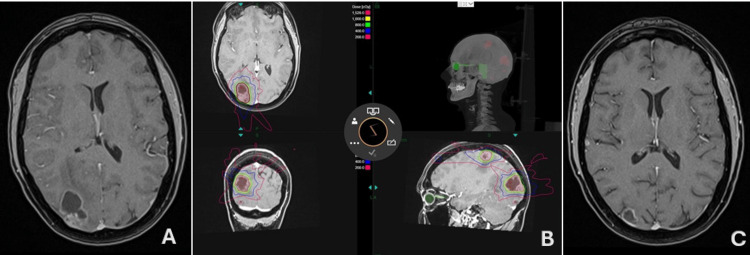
Patient C, pre- and post-GRS imaging (A) Pre-treatment axial T1 post-contrast MRI of metastatic lesions. (B) Staged GRS protocol treatment planning for two large occipital and parietal metastases. PTV of 19.3 cc treated in two fractions with a prescription dose of 20 Gy, delivered at 58.9% isodose line. (C) Post-treatment axial T1 post-contrast MRI. GRS: gyroscopic stereotactic radiosurgery
